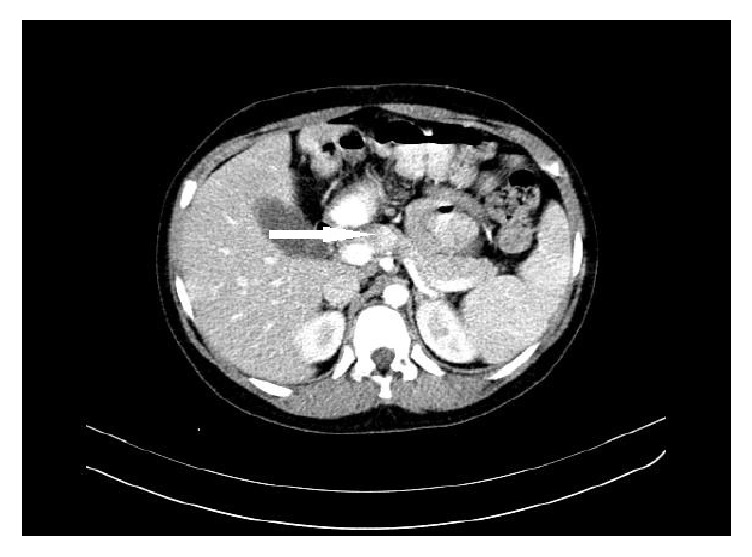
Abdominal CT scan with enhanced mass at the neck of pancreas (arrow).
